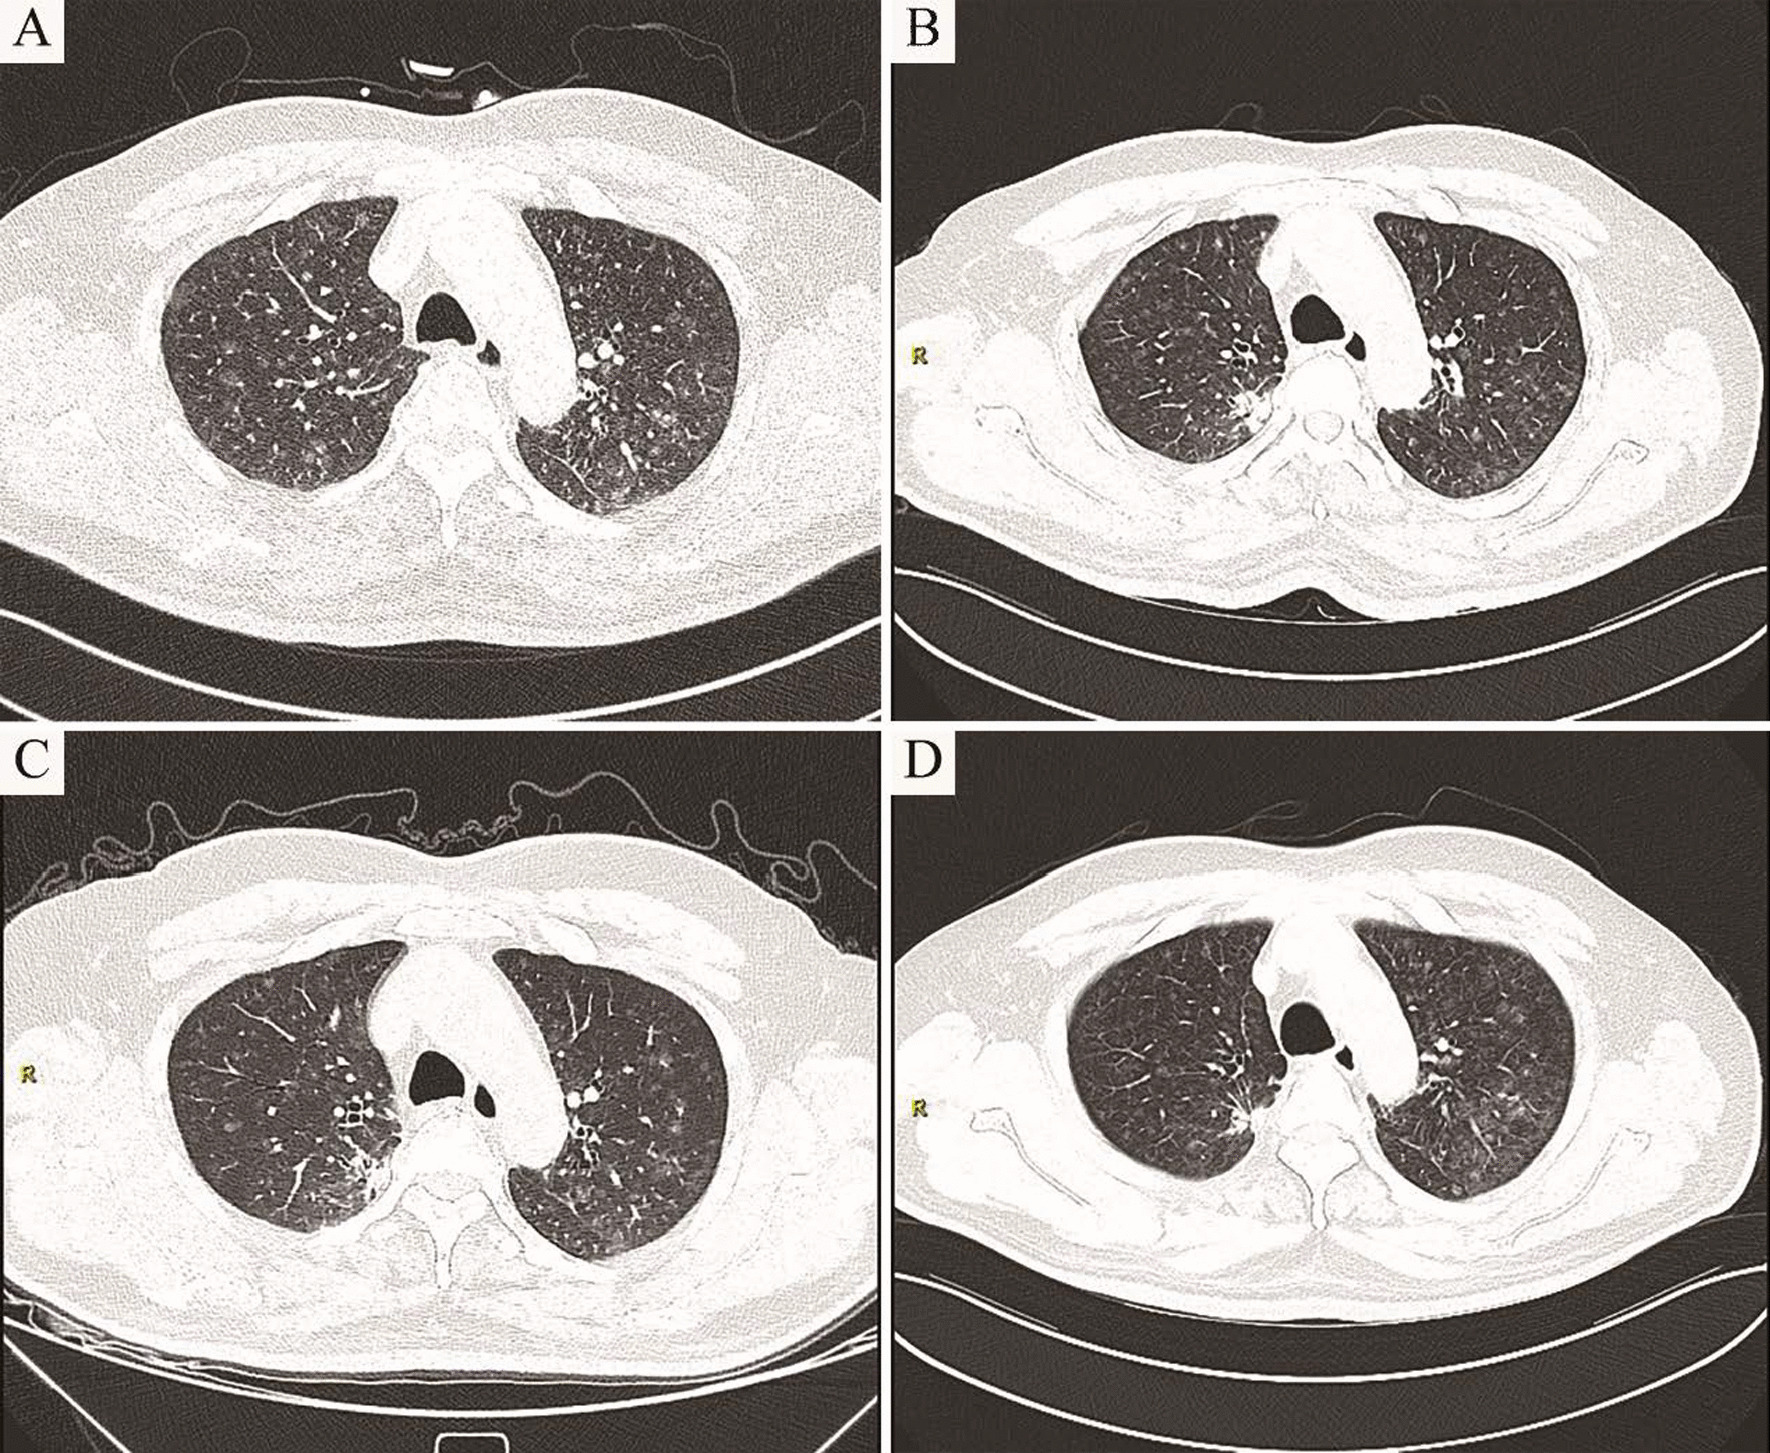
Imaging changes on Computed tomography. a HRCT showed multiple randomly distributed ground glass nodules in the center of the lobules throughout the lung fields, particularly in the upper lungs (2018-05-23). b HRCT after three months of follow up showed no significant change in GGOs in the bilateral lungs (2018-09-03). c HRCT after follow up for half a year revealed no significant change in GGOs (2018-12-13). d HRCT after follow up for a year revealed no significant change in GGOs (2019-07-08)
